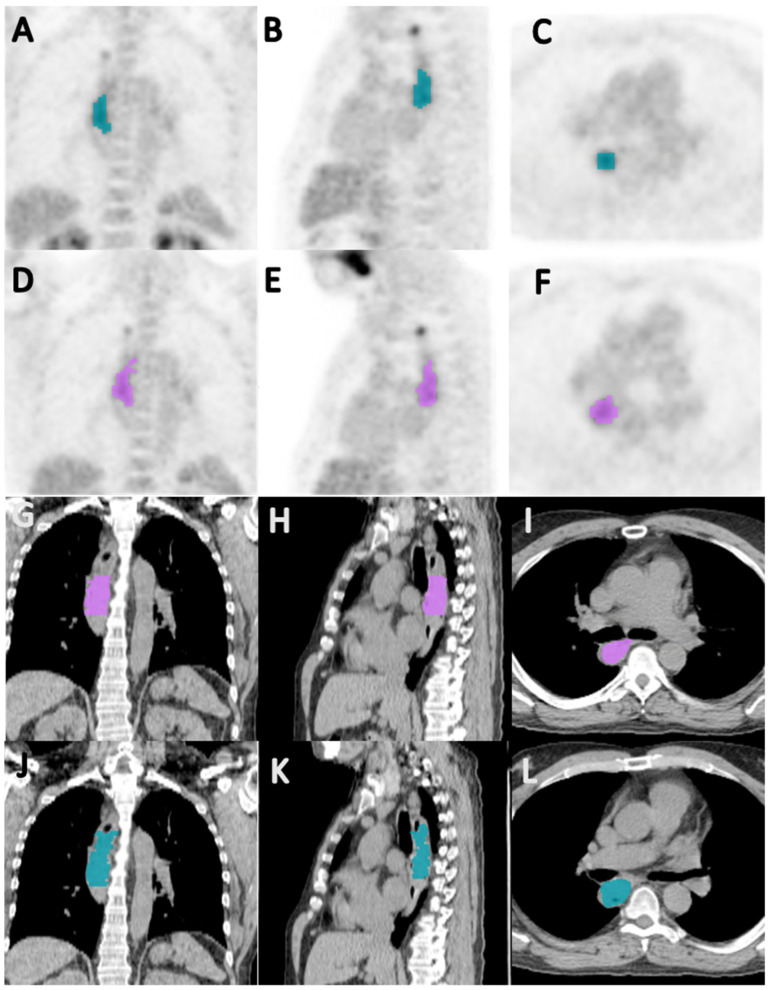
Coronal (A,D), sagittal (B,E), and axial (C,F) PET images, and coronal (G,J), sagittal (H,K), and axial (I,F) CT images showing segmentation of primary tumor by reader 1 (A–C,G–I) and reader 2 (D–F,J–L), in a 64-year-old male patient with esophageal squamous cell carcinoma; the Jaccard index and a dice similarity coefficient for inter-reader agreement on the segmented volumes were 0.657 and 0.793, respectively.
